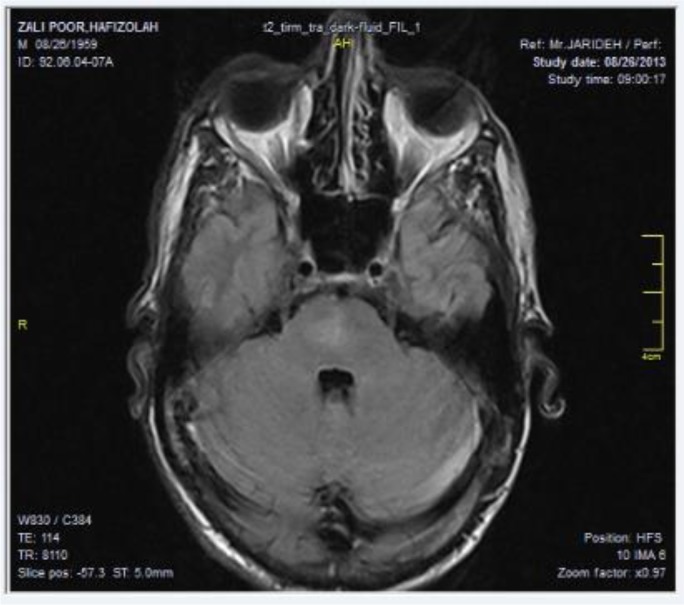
MRI image (FLAIR) revealed an increase in signal intensity of pons
